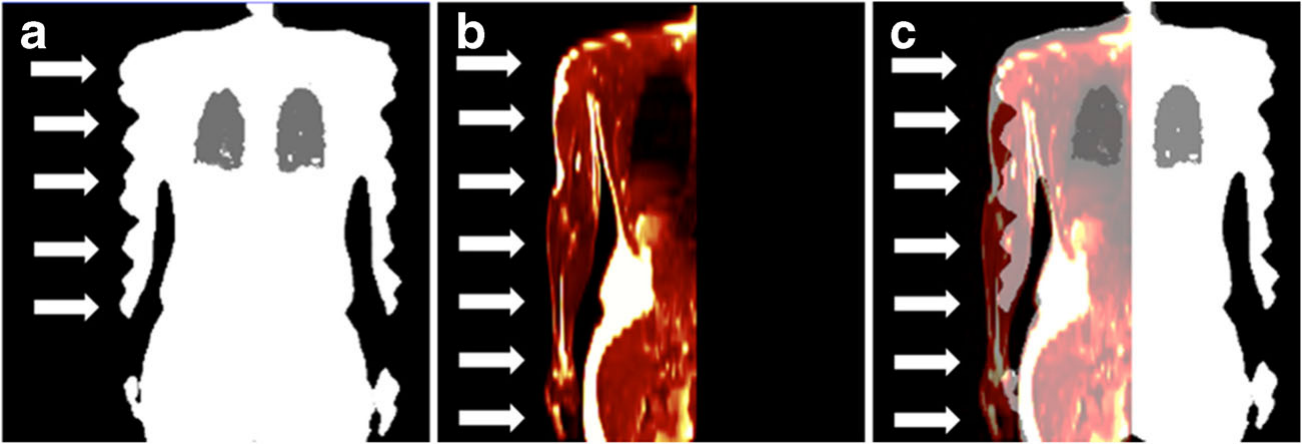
Truncation artefact correction in hybrid PET/MRI imaging. The FOV in MR imaging is limited, leading to signal truncations in the MR-based attenuation map (A, *arrows* along the arms). These signal truncations cause an attenuation bias during attenuation correction (under-correction). The HUGE technique increases the FOV in MR imaging eliminating these artefacts (B, *arrows*). The fusion of truncated attenuation map (A) and the HUGE images (B) shows this effect (C)
